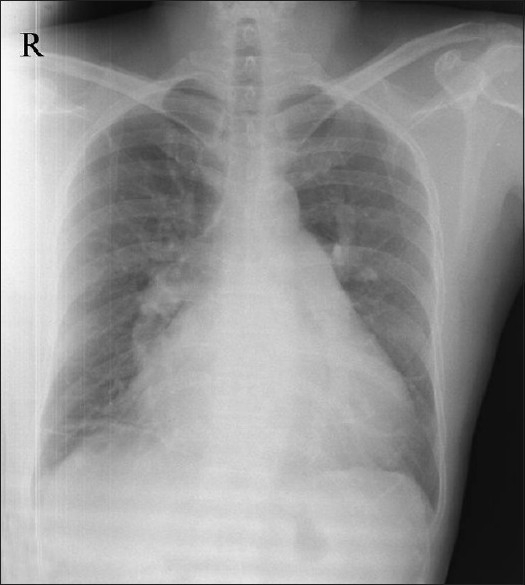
X-ray chest in postero-anterior view showing cardiomegaly and increased pulmonary blood flow, dilated right atrium and dilated pulmonary artery
